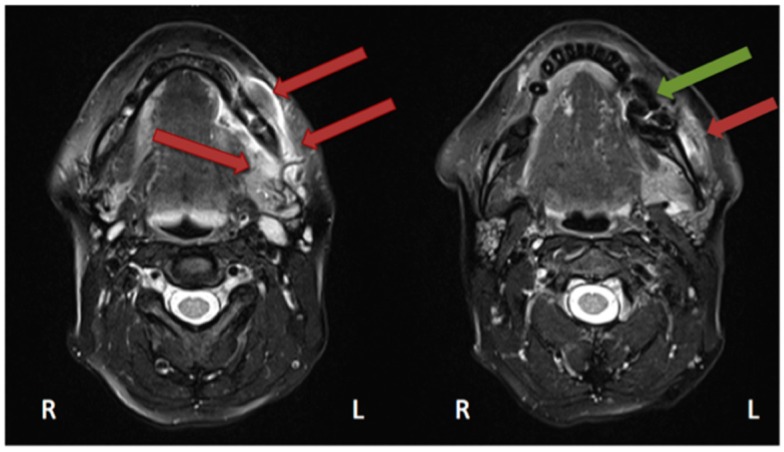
Magnetic resonance imaging (Siemens, Avanto, 1.5T, Sequence: T2 tse tra) Patient: 48 years old, female, metastatic breast cancer, zoledronic acid for 2 years (panoramic radiograph Figure 1). Green arrow showing the MRONJ necrosis: hypointense bone marrow, red arrows showing the oedema. Pair of screenshots. AAMOS staging: stage 2.
